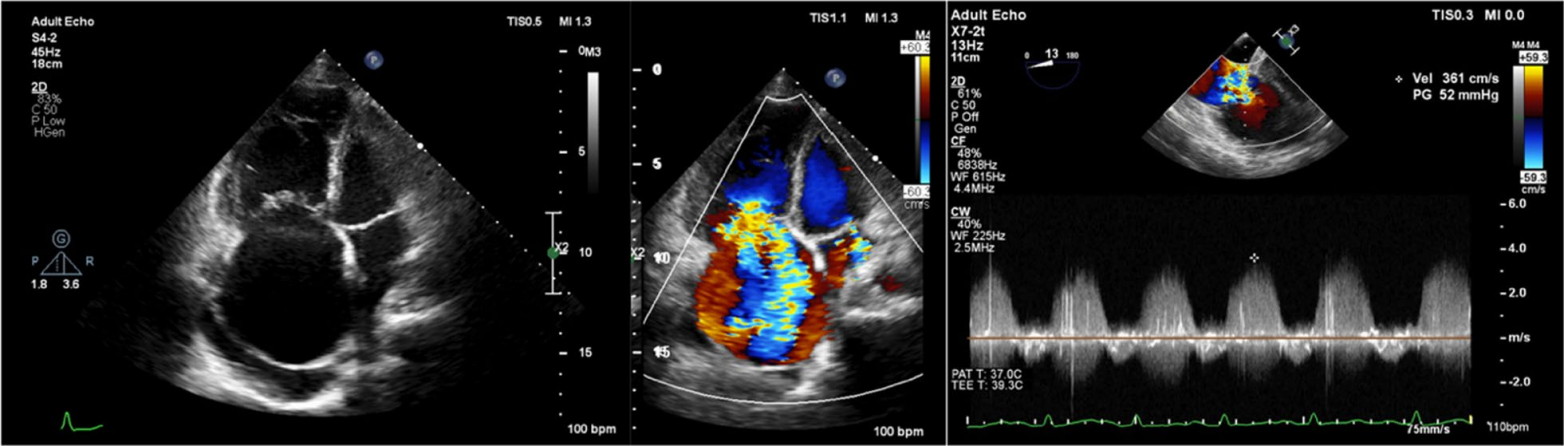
Echocardiography showed dilation of the right atrium and ventricle, bulging IAS to the left atrium, and severe tricuspid valve regurgitation
